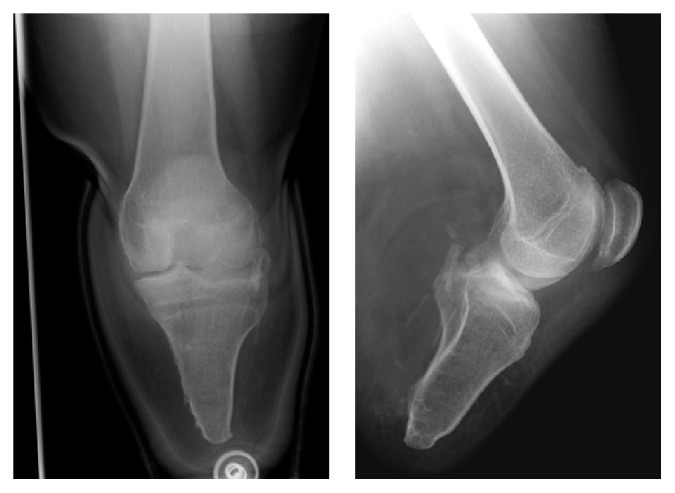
AP and lateral radiographs showing below-knee amputation stump with relative patella alta.
